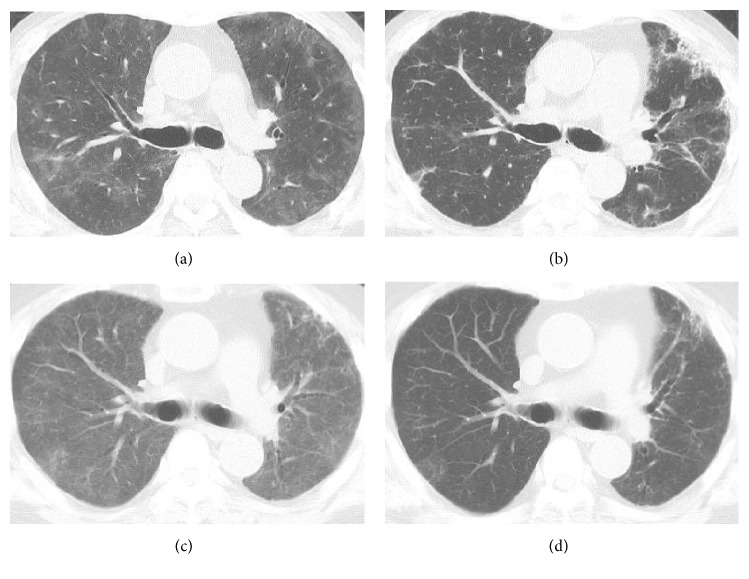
Computed tomography of the chest at the first time appearance of dyspnea (a), after treatment with prednisone 40 mg/day (b), at recurrence of dyspnea (c), and after treatment with methylprednisolone pulse and cyclophosphamide pulse therapy (d).
